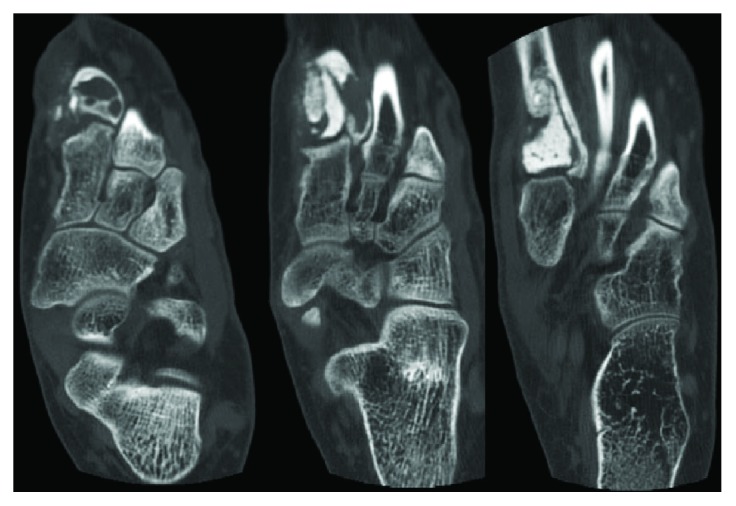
Computed tomography (CT) scan demonstrating an expansile lucency at the proximal aspect of the first metatarsal base toward the dorsal lateral side.
